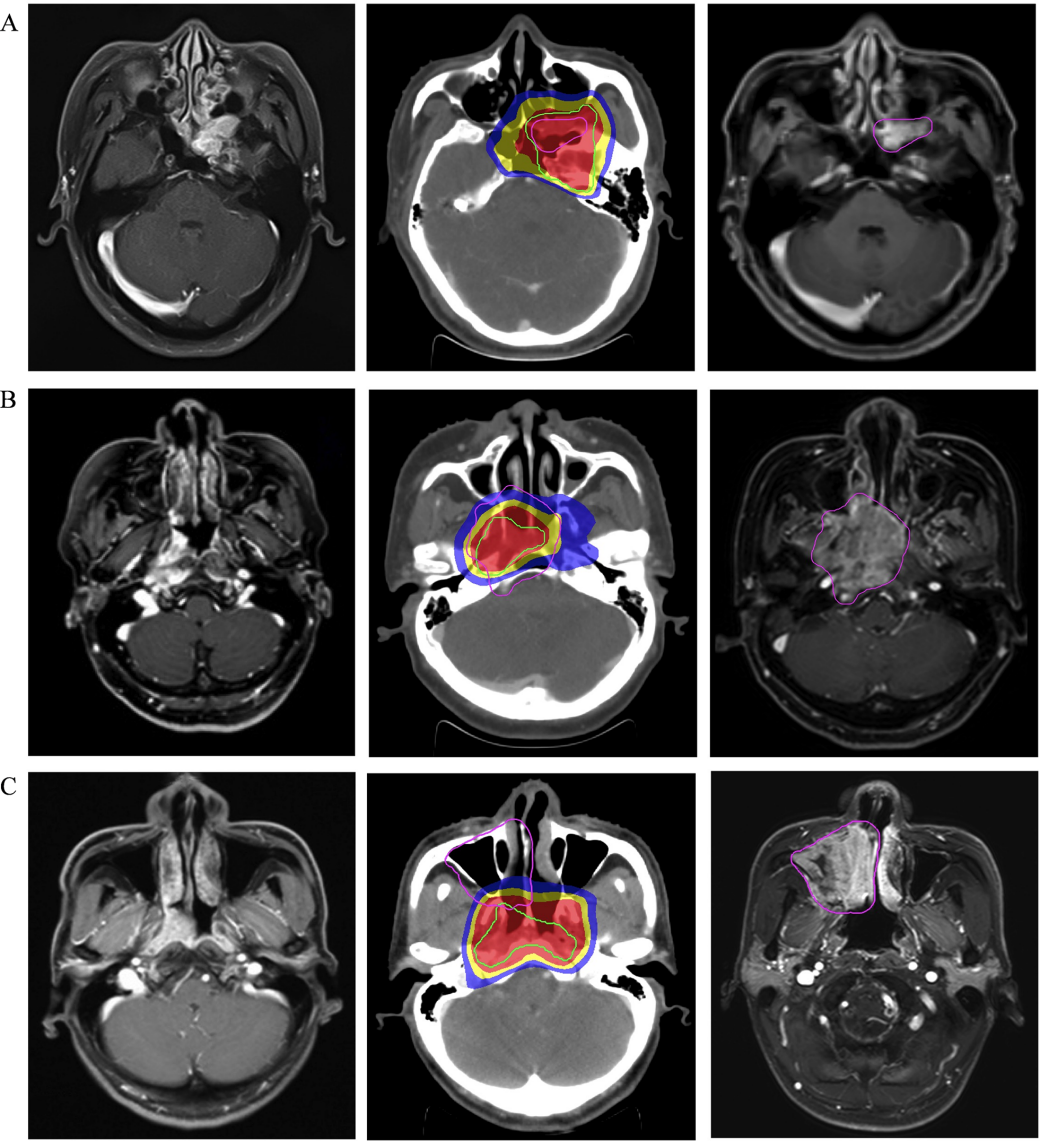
Three different types of recurrent patterns of NPC treated with IMRT. a in field failure; b marginal failure; c outside field failure. Left, pretreatment magnetic resonance imaging (MRI); Middle, the recurrent tumor was transferred from the MRI at the time of recurrence to the planning computed tomography (CT) to present doses to the recurrent sites; Right, MRI at the time of recurrence. The green line represented the initial gross target volume; The pink line represented the recurrent tumor volume; The red color-wash represented 70 Gy, yellow represented 66 Gy, and blue represented 60 Gy
